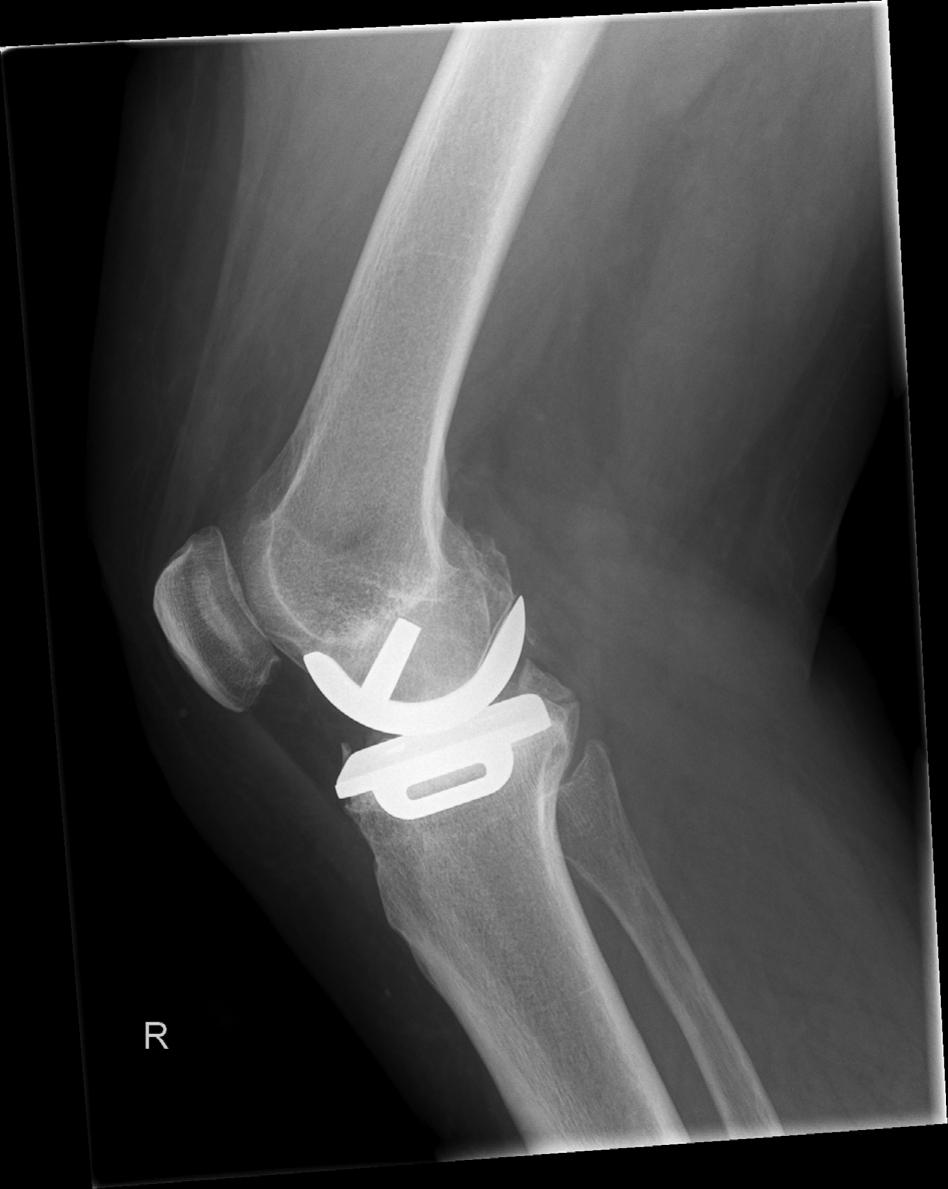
Preoperative lateral radiograph showing the unicompartmental knee arthroplasty.
